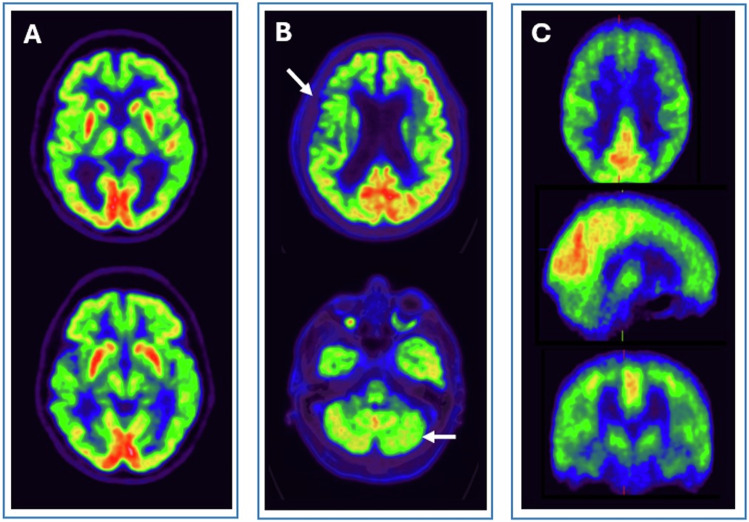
Pitfalls and artefacts in [^18^F]FDG PET imaging. A Scan of a patient with CNS drug interference showing cortical hypometabolism that could mimic an AD pattern, but with thalamic hypometabolism characteristic of this interference. B Scan of a patient with a right frontoparietal ischaemic antecedent (arrow), showing a diaschisis of the contralateral cerebellum (arrow) due to disruption of the corticospinal tract. C An example of a PET scan with motion artefacts
